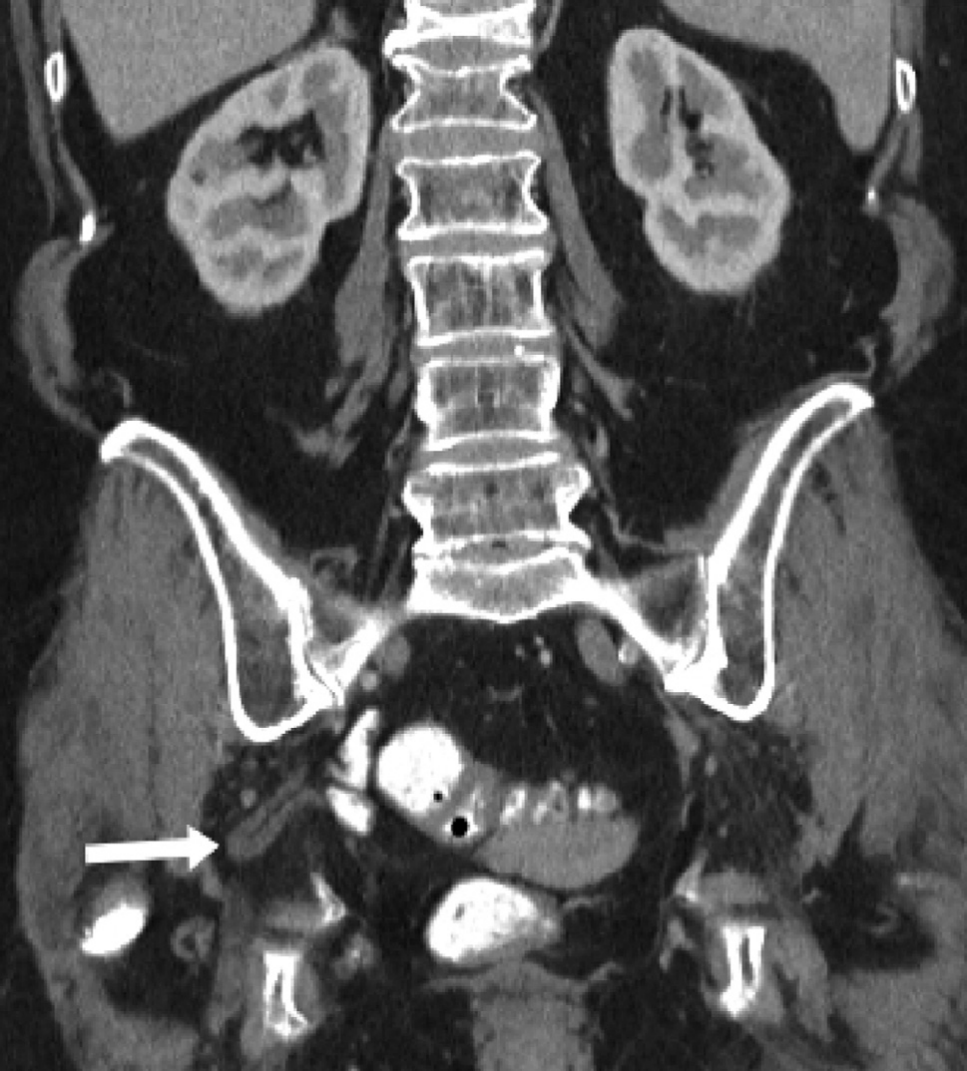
Coronal CT image in the nephrographic phase demonstrates a right ureterosciatic hernia with looping consistent with the curlicue sign (white arrow). Chronic compression fractures of the T12 and L1 vertebrae are also present.
